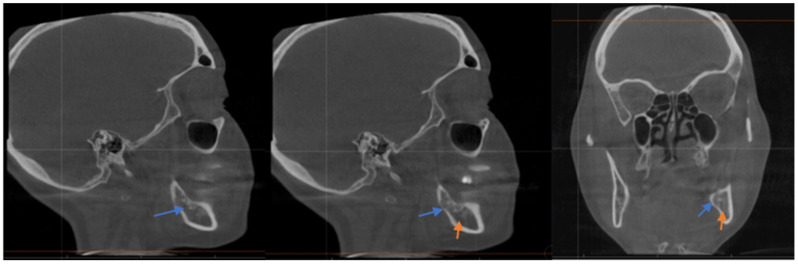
Sagittal CT scan: Note the proximity of the mineralized xenograft in tooth #17 (Blue Arrow) following surgical removal and bone graft procedure to inferior alveolar nerve canal (Orange Arrow).
